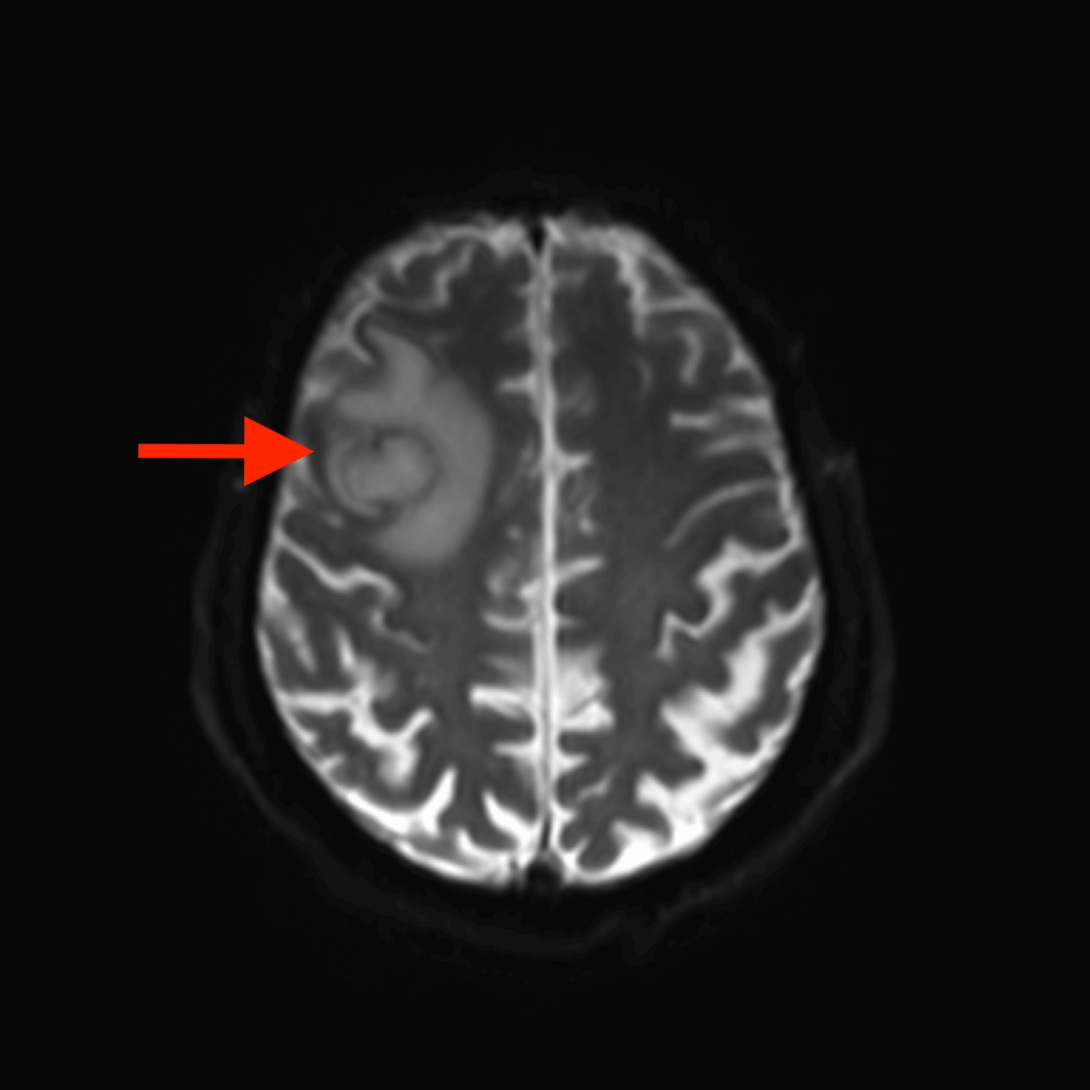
MRI of the brain showing a right frontal lesion with restricted diffusion
